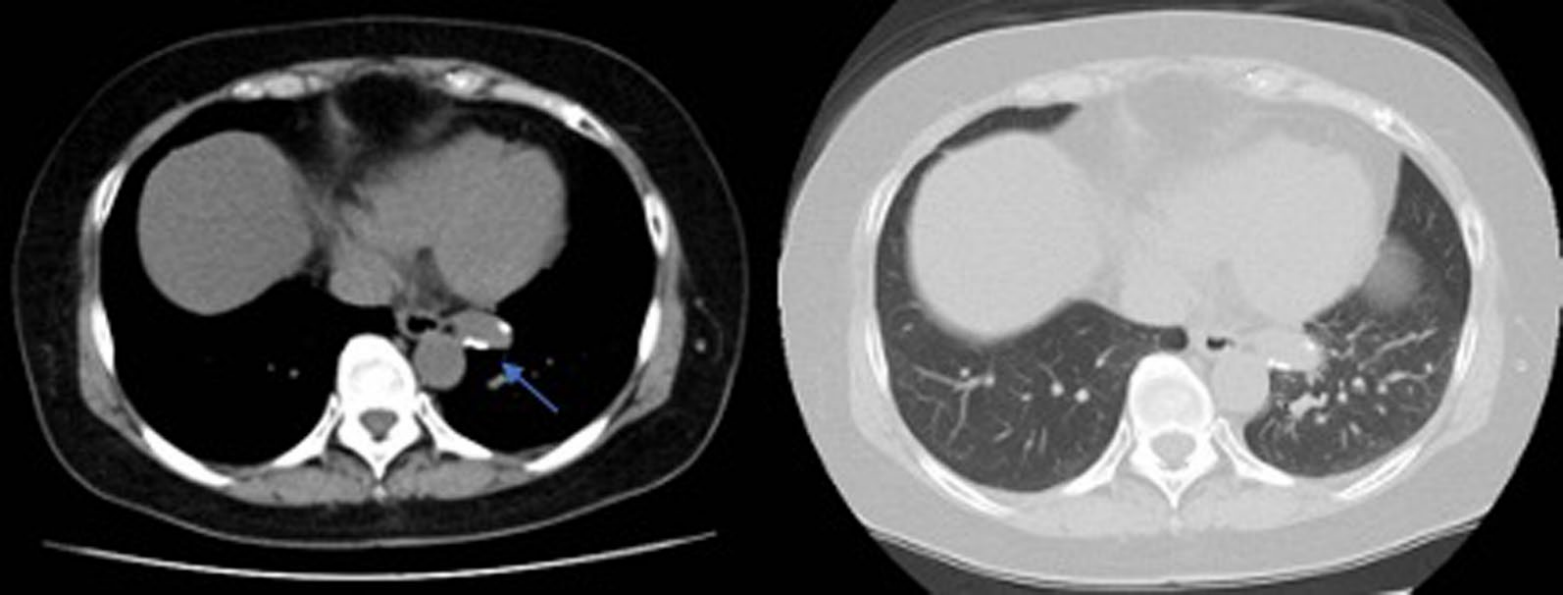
CT of the chest showing the abnormal artery with calcification(arrow)
